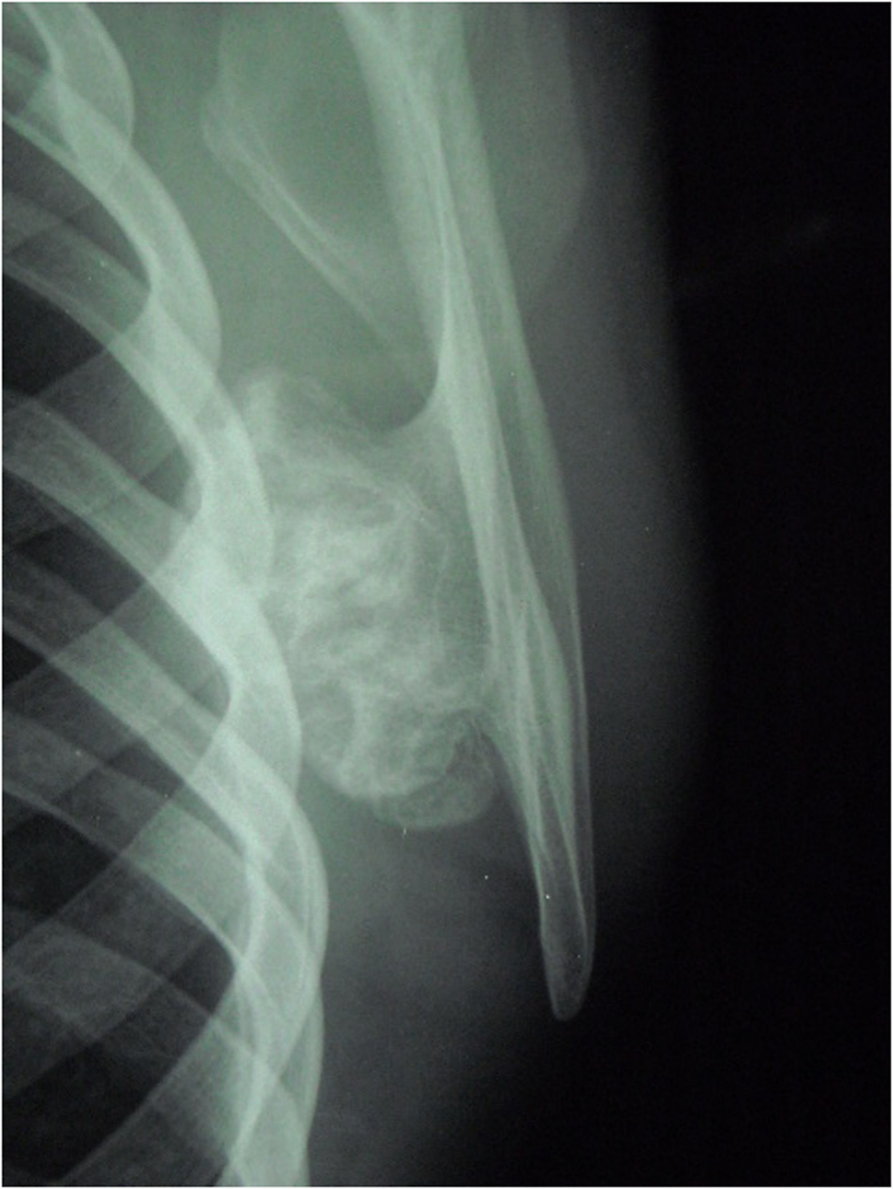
Radiographic study showed a large bony lesion deforming the medial border of the left scapula.
